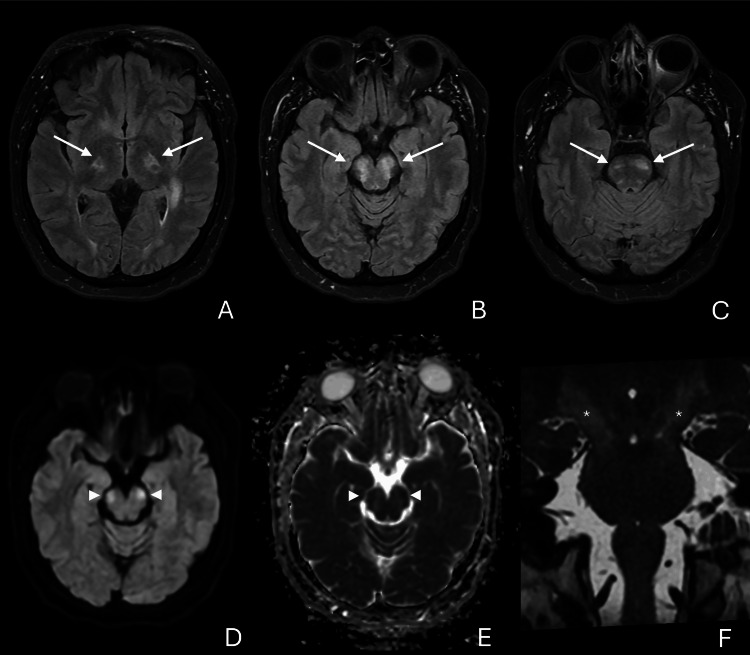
Brain MRI findings (A–C) Axial T2-weighted fluid-attenuated inversion recovery (FLAIR) images demonstrate bilateral hyperintensities along the corticospinal tracts (white arrows), indicative of tract-specific involvement; (D–E) Diffusion-weighted imaging (DWI) shows areas of restricted diffusion (arrowheads), with corresponding hypointensity on the apparent diffusion coefficient (ADC) map, consistent with cytotoxic edema in acute lesions; (F) Coronal T2-weighted sequence further delineates tract involvement, showing longitudinal hyperintensity extending from the posterior limb of the internal capsule through the cerebral peduncles to the pontine base (asterisks), tracking the full course of the corticospinal tracts.
